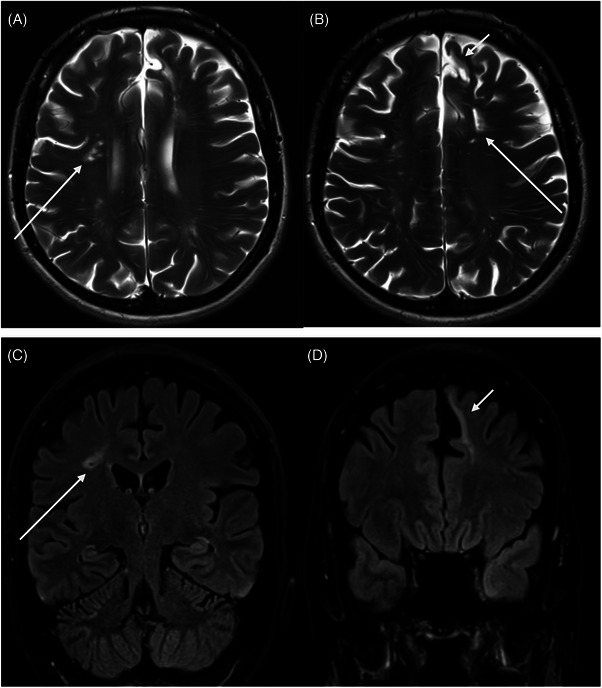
Cerebral magnetic resonance imaging of patients with antiphospholipid syndrome showing infarction. Deep watershed and separate cortical embolic infarcts by (A and B) T2 axial and (C and D) 2D fluid attenuated recovery sequence (FLAIR). Long arrows demonstrate deep watershed hyperintense foci (A and B) which were subsequently supressed on FLAIR imaging, confirming gliotic change secondary to infarction (C). Short arrows outline cortical infarction of the left anterior cerebral territory within the superior frontal gyrus (B and D).
